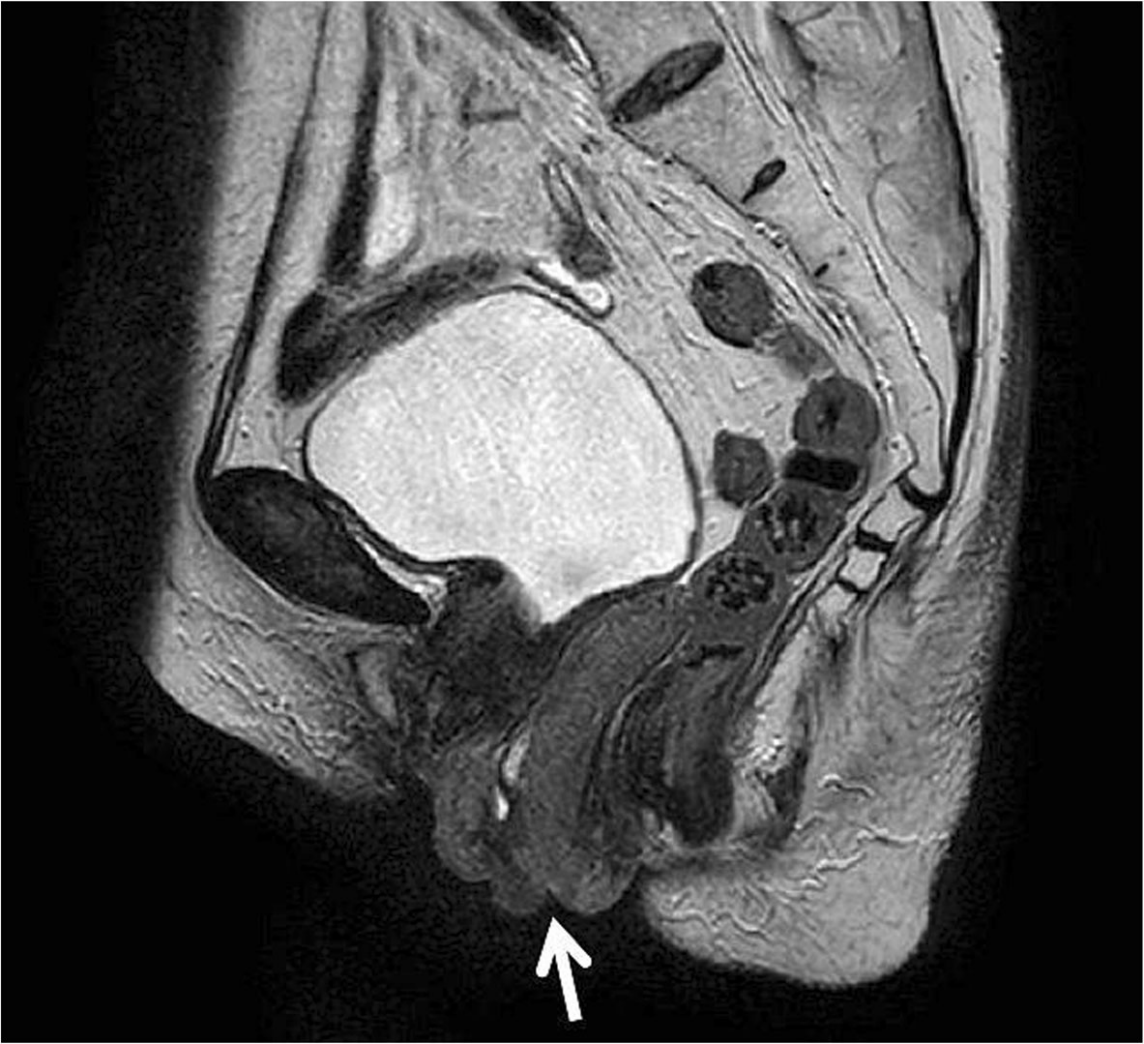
T2-weighted magnetic resonance imaging in the sagittal plane showing a stage IV completely prolapsed uterus defined as eversion of the total length of the lower genital tract. The external cervical os (white arrows) was observed, but no endometrial cavity was detected
